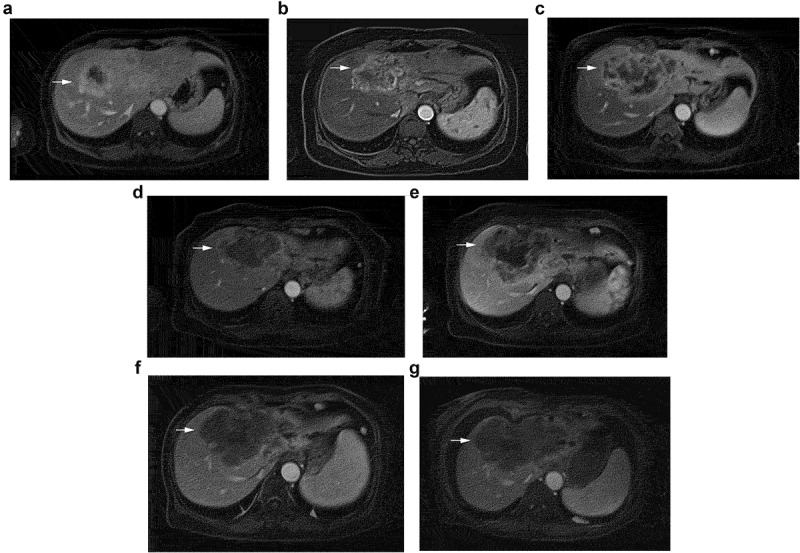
The change of enhanced computed tomography of liver.
